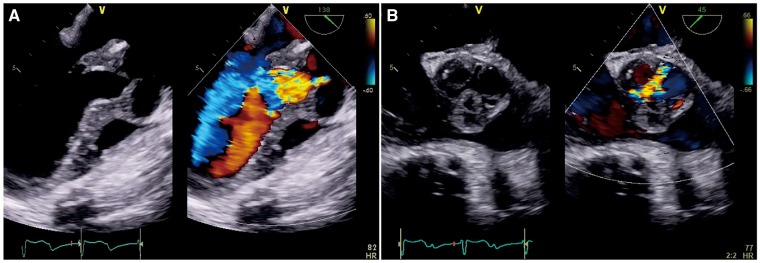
Transoesophageal echocardiography showing the thickened aortic valve with severe aortic regurgitation (*A*, aortic valve long-axis; *B*, aortic valve short-axis).
